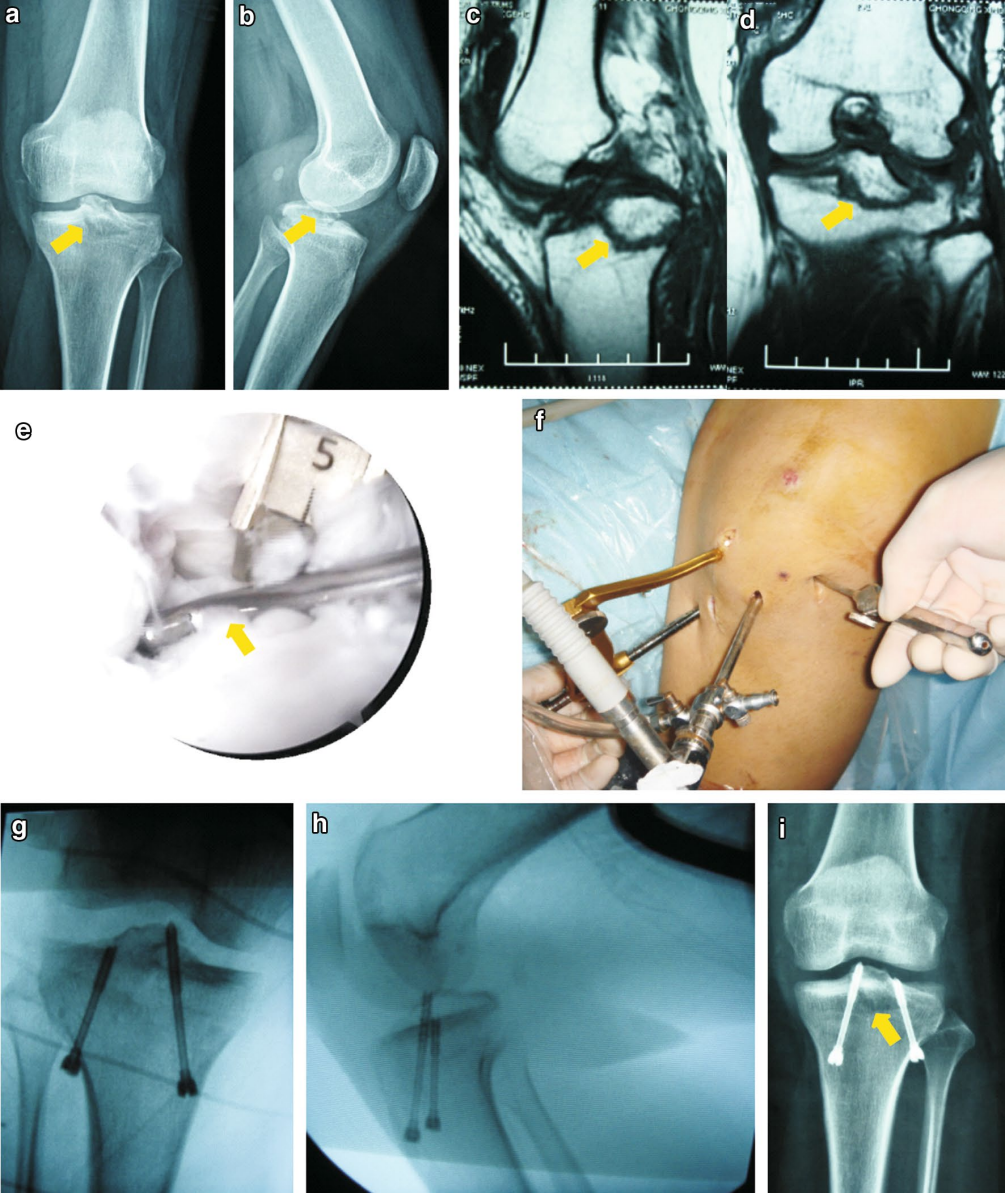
PCL avulsion fracture of a 32-year-old female was fixed anteriorly under arthroscopy with two partially threaded cannulated screws. a, b X-ray and CT showed the tibial intercondylar eminence fracture. c, d MRI confirmed the fragment size and displacement of the PCL avulsion fracture. Fragment involved the medial and lateral tibial plates. e, f Standard knee arthroscopy was performed with anterolateral and anteromedial portals using a 30° 4.0 mm arthroscope. The tibial PCL guide (acufex) and the probe were used to secure the fracture fragment and guide kirschner wires. g–i The position of two partially threaded cannulated screws was confirmed under image intensifier and X-ray examination after operation. *Arrow* pointed the fracture
